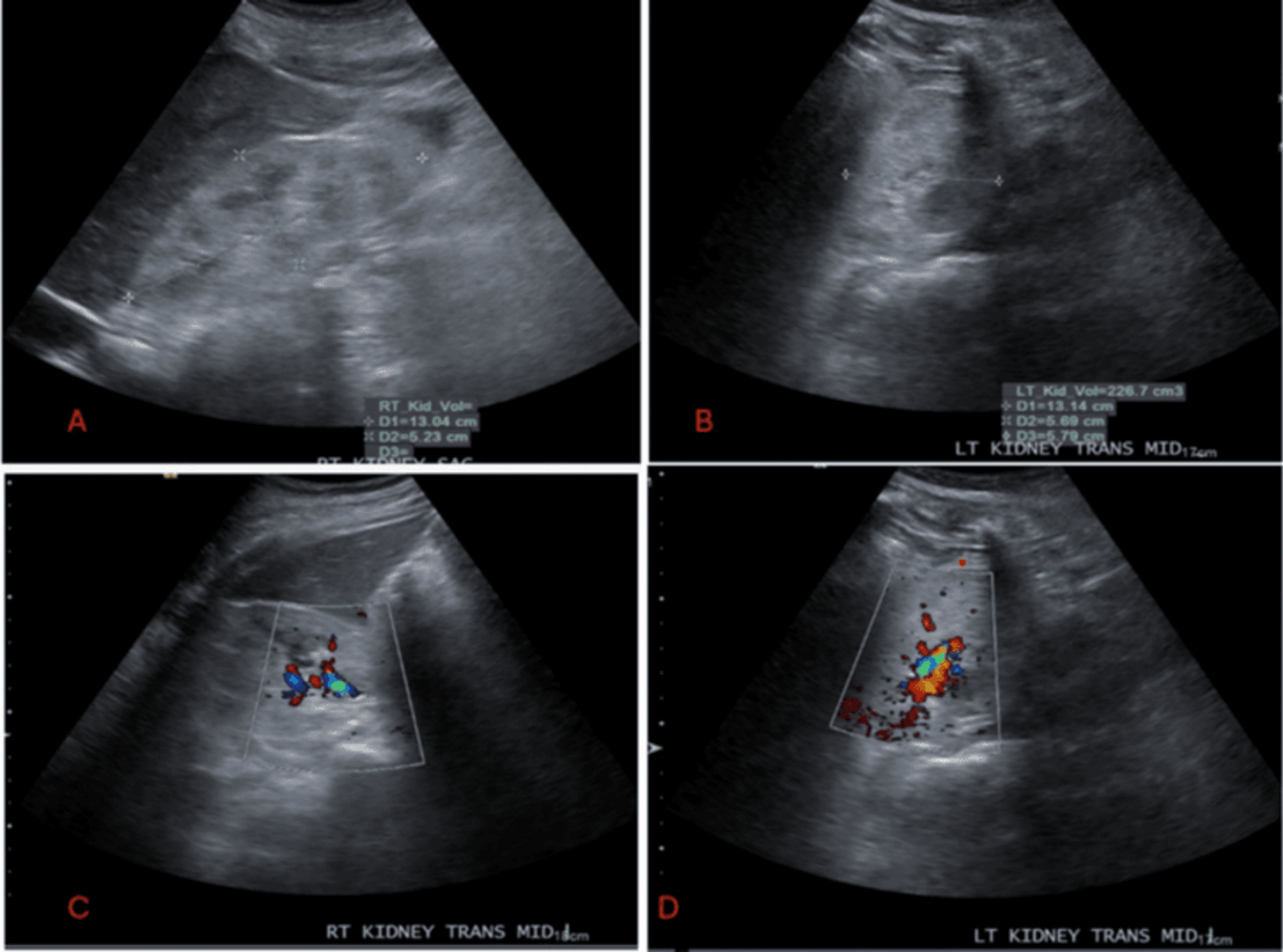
Kidney ultrasound images A. Right kidney with size 13.0 x 5.2 x 6.7 cm, normal parenchyma thickness and contour but Increased echogenicity. No Pelvicalyceal dilatation, calculi, cysts, or solid masses B. Left kidney with size 13.1 x 5.7 x 5.8 cm, normal parenchyma thickness and contour but increased echogenicity. No pelvicalyceal dilatation, calculi, cysts, or solid masses C. Doppler ultrasound of the right Kidney showing normal perfusion. D. Doppler ultrasound of the left Kidney showing normal perfusion
